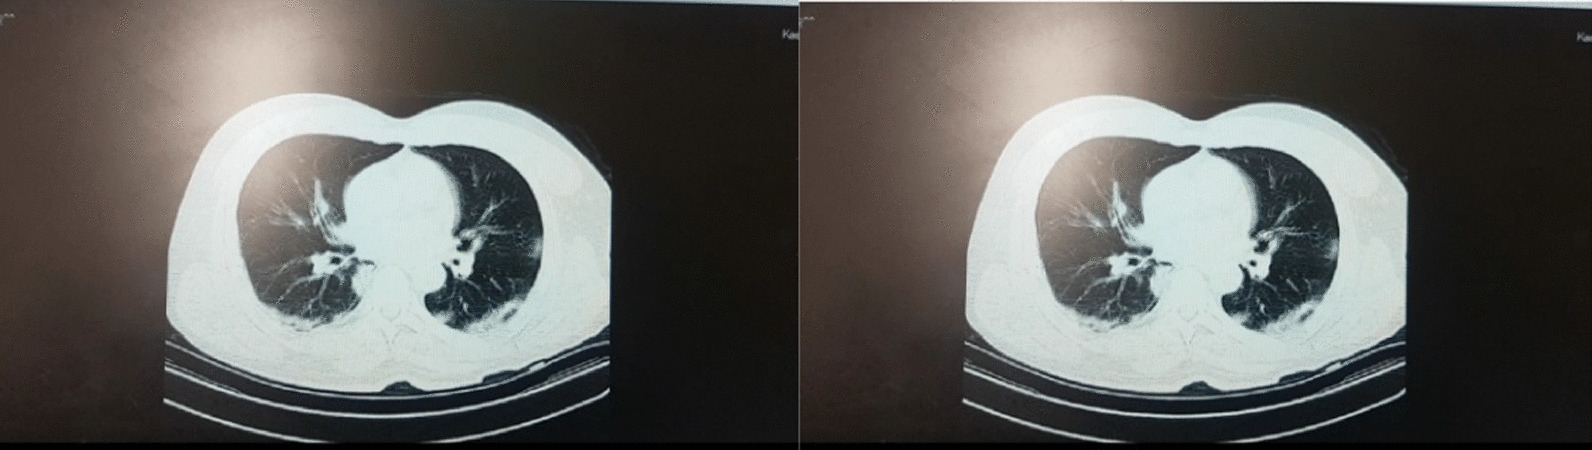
Lung high-resolution computed tomography
